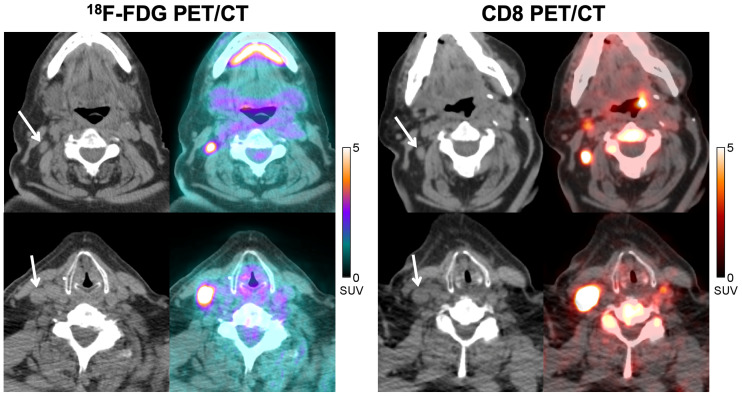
A 77-y-old man with metastatic melanoma treated with pembrolizumab. CT and fused ^18^F-FDG PET/CT images (left) acquired at approximately 8 mo after initiation of immunotherapy demonstrate 2 ^18^F-FDG–avid nodal metastases in right neck (SUV_MAX_ = 8.0, top image; SUV_MAX_ = 16.8, bottom image), which could represent viable metastases. Corresponding CT and fused CD8 PET/CT images (right) obtained at 1 mo after ^18^F-FDG PET/CT demonstrate significant tracer activity in both metastases (SUV_MAX_ = 5.4, top image; SUV_MAX_ = 14.6, bottom image), which suggests that some of the ^18^F-FDG activity could be due to tumor-infiltrating CD8+ T cells rather than tumor cells. Follow-up imaging over the next 6 mo demonstrated stable disease, supportive of this hypothesis.
